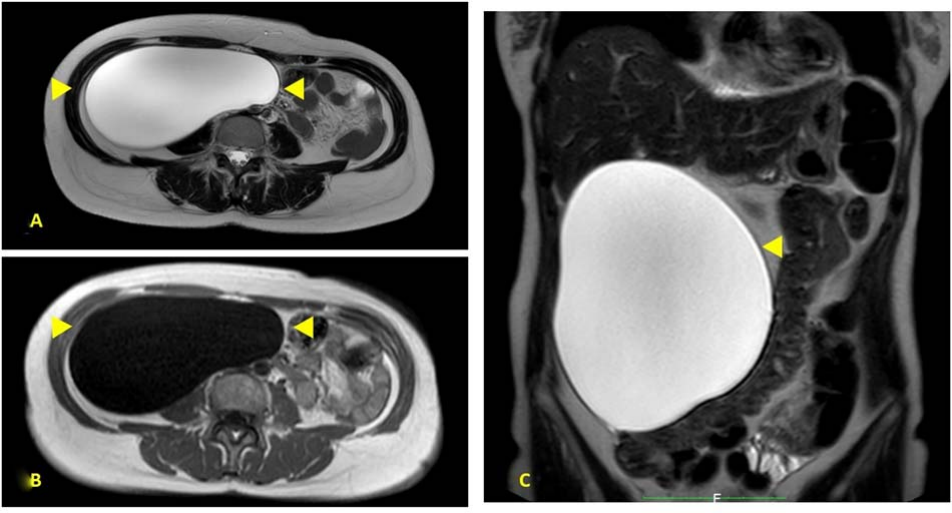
(A) Prohance® MR; main lesion appears hyperintense on T2-weighted sequences (arrowheads); (B) main lesion appears hypointense on T1-weighted sequences (arrowheads); (C) coronal T2-weighted sequences confirmed main radiological findings: size 15 × 20 cm, thin aspect of envelope, absence of infiltrative aspect and gross displacement of surrounding structures.
